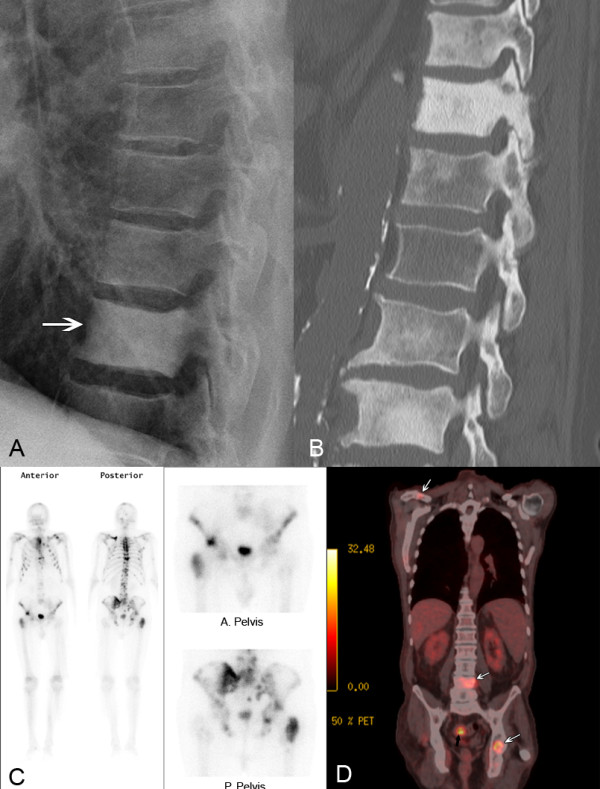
Skeletal Metastasis. A. A conventional radiograph shows osteoblastic deposition within the vertebral bodies of this 62 year old man with metastasis from prostate carcinoma (arrow). B. A sagittal CT image in another patient reveals extensive osteoblastic involvement throughout the lumbar and thoracic spine in addition to aortic atherosclerosis. C. A nuclear medicine planar bone scan in another patient with prostate carcinoma shows multiple areas of increased uptake within the bones of the axial skeleton. Bone scans are the most widely used primary imaging examination for detecting osseous metastasis. Because bone scans are non-specific, areas of abnormality should be followed up with radiographs, CT or MRI to specifically confirm the pathology. D. In another patient with colorectal carcinoma, a FDG-PET, fluorine-18-2-fluoro-2-deoxy-D-glucose positron emission tomography fused with CT shows intense uptake at the site of primary bowel carcinoma (black arrow) as well as several sites of osseous metastasis in the left acetabulum, right clavicle, and L4 vertebral body (white arrows). (Images courtesy of Lindsay J. Rowe, Newcastle, Australia).
